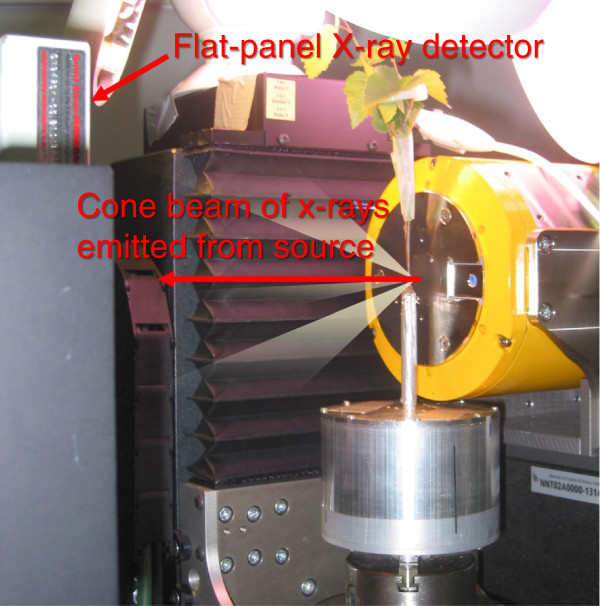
The XMT setup. X-rays are emitted in a wide cone beam from the x-ray tube on the right, and detected with the flat-panel detector to the left. Only the upper corner of the detector is visible from behind its mounting.
